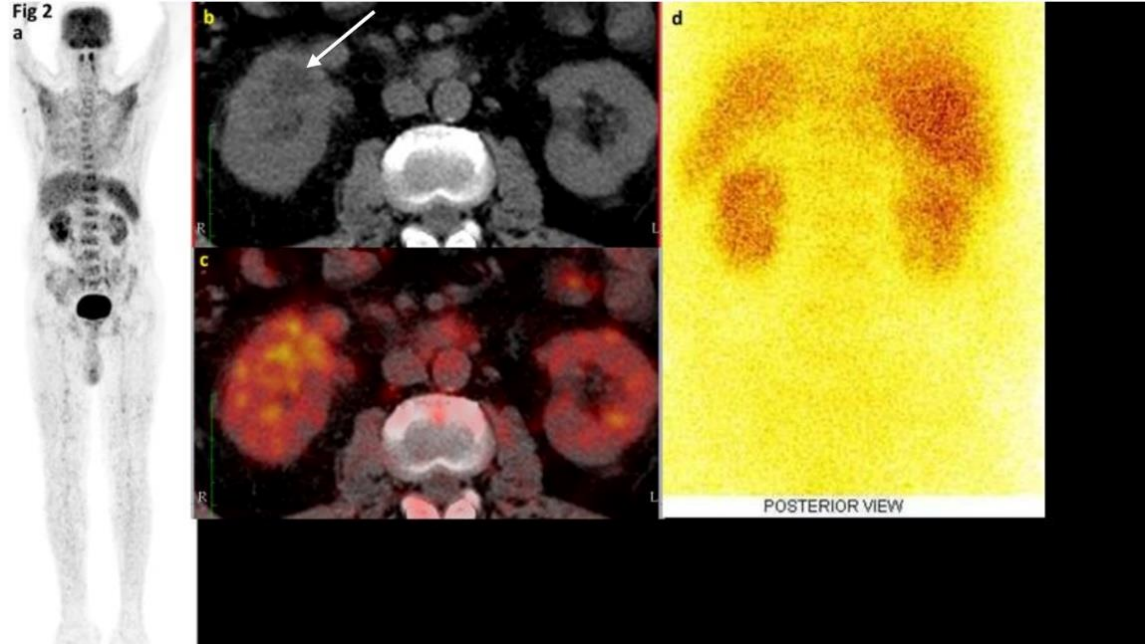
A 65-year-old male with pyrexia undergoing FDG PET/CT for occult infection (Scan showed a focus of hypodensity in the right kidney [b] that showed increased FDG uptake [c]. ^99m^Tc-DMSA scan showed irregular contour of both kidneys suggesting scars [d])
